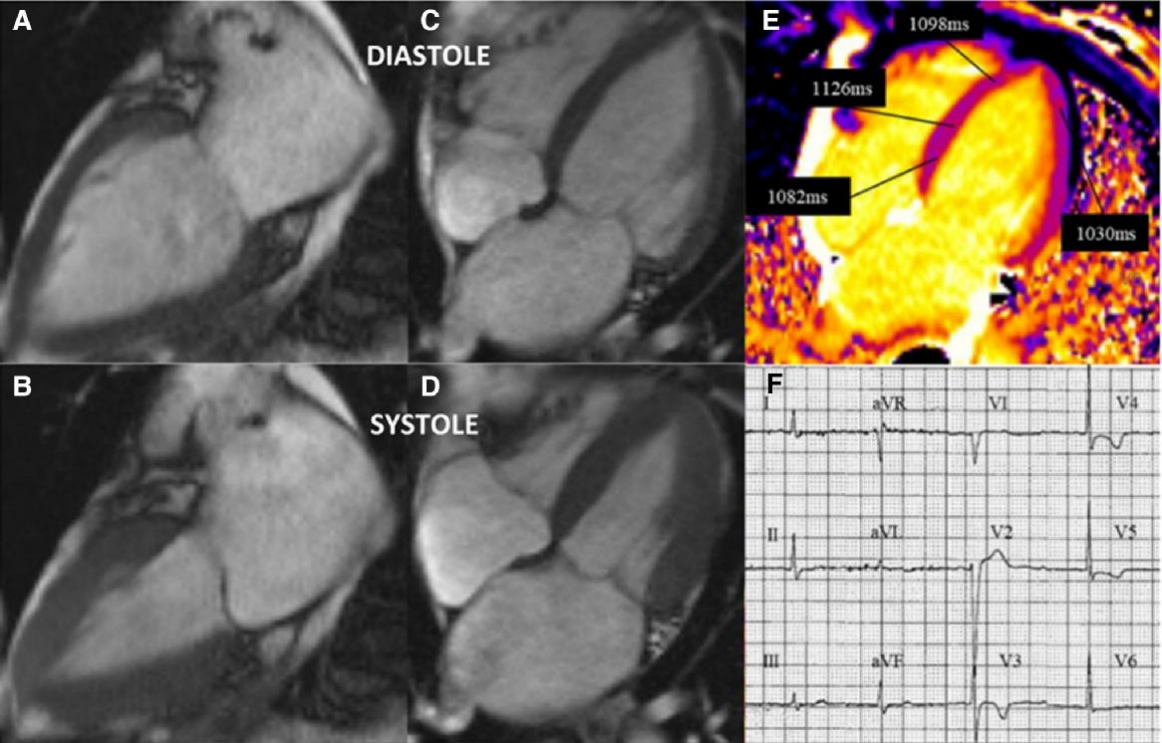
Cardiac MRI at 15 months. Complete resolution of apical wall thickening, (*A*, *C*) diastole, (*B*, *D*) systole. Resolving T1 times, (*E*) and persisting T-waves changes (*F*).
